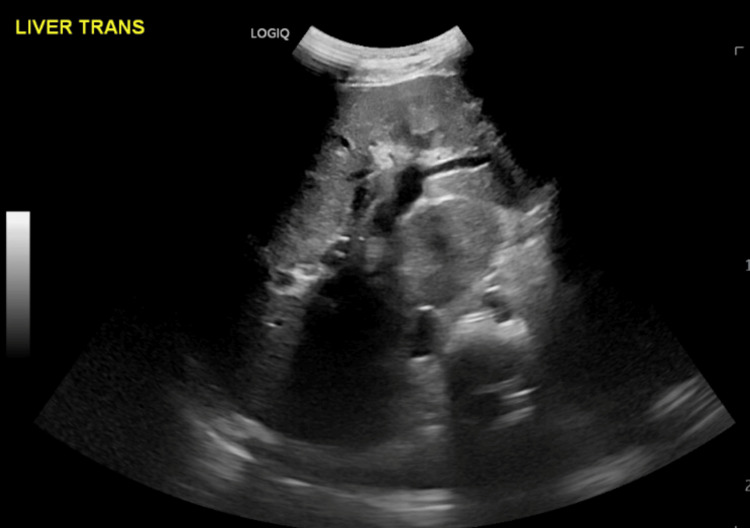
Ultrasonographic evidence of biliary tree dilatation, indicative of primary sclerosing cholangitis
